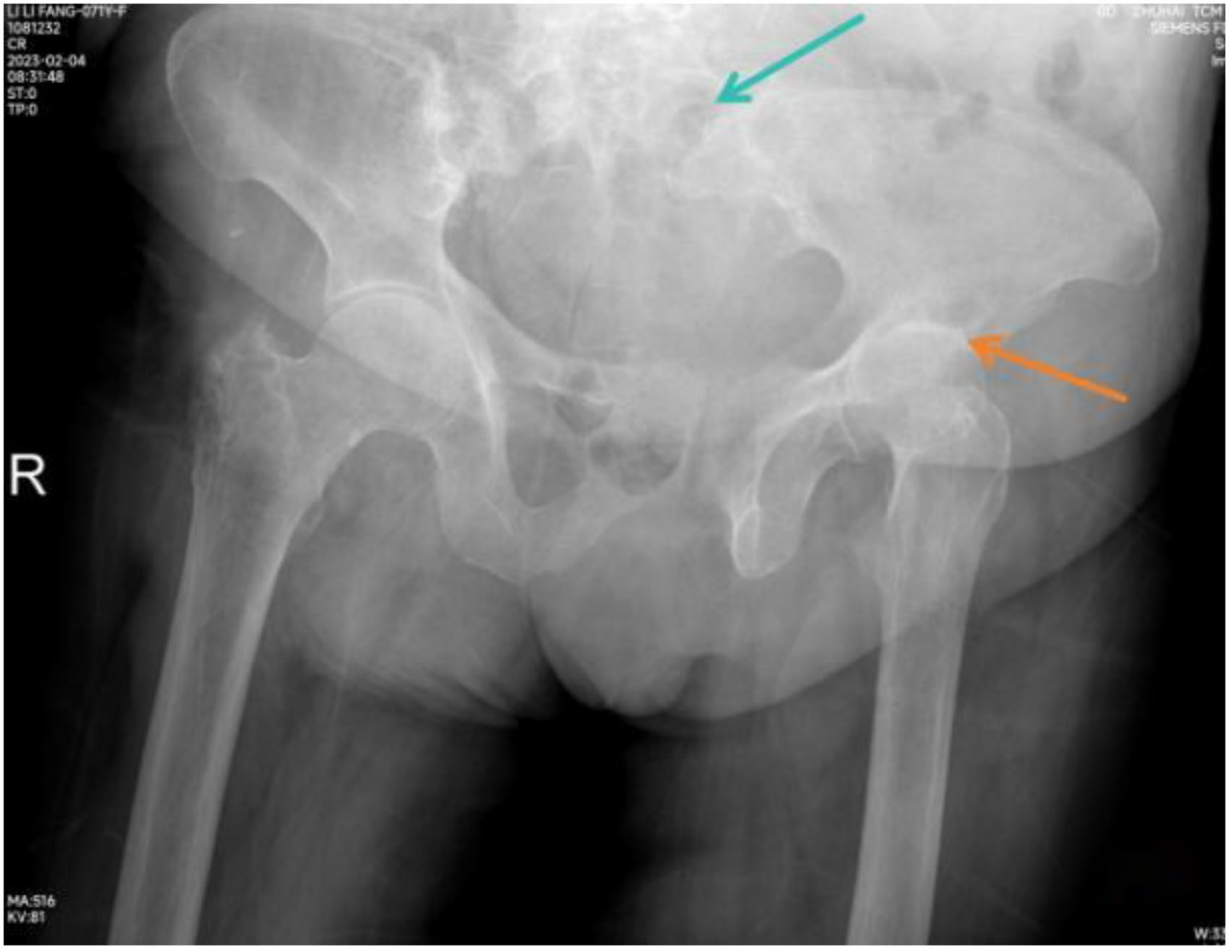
Plain pelvic radiographs showed erosion of the sacral and iliac borders with widening of the left sacroiliac joint (chrysanthemum blue arrow), space narrowing, low-density cystic lesion of left hip (orange arrow).
